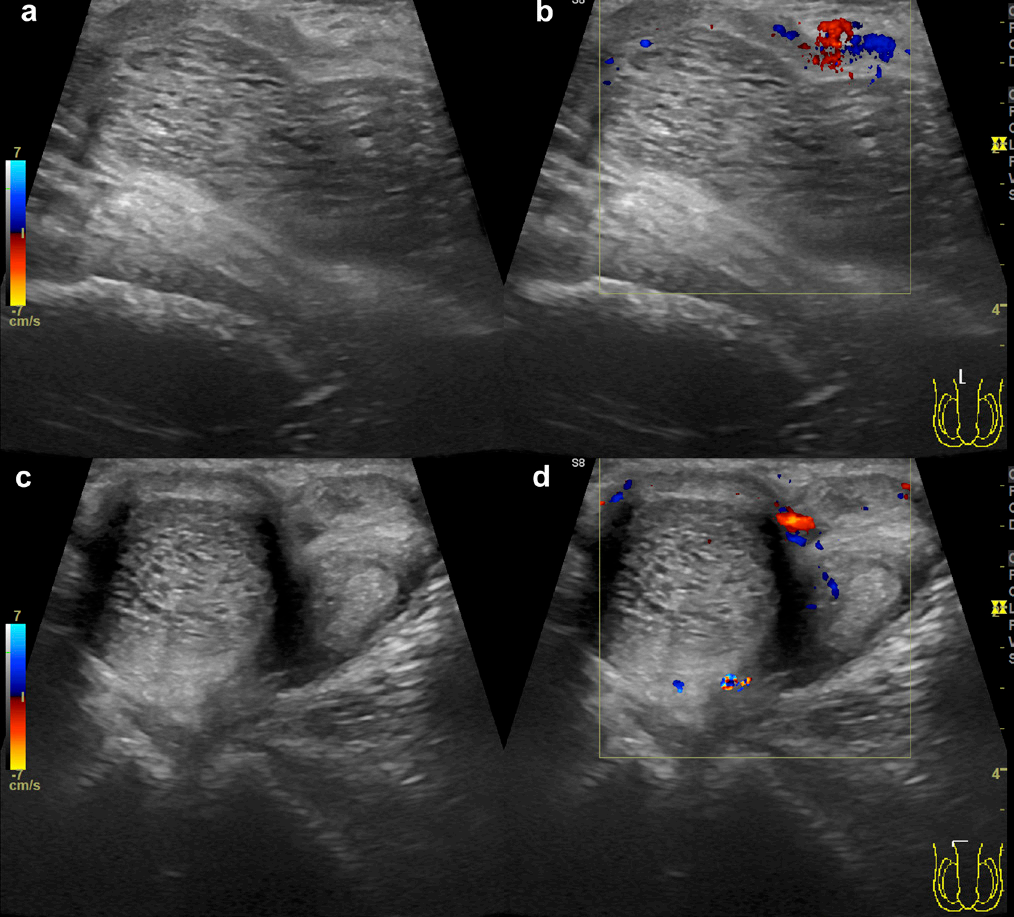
Ultrasound (2a, 2c) at the base level of the penis revealed a well-circumscribed right-sided corpus cavernosum, enlarged and round-shaped, with heterogeneous decreased echogenicity. Doppler ultrasound (2b, 2d) showed no vascularity inside the abnormal image.
